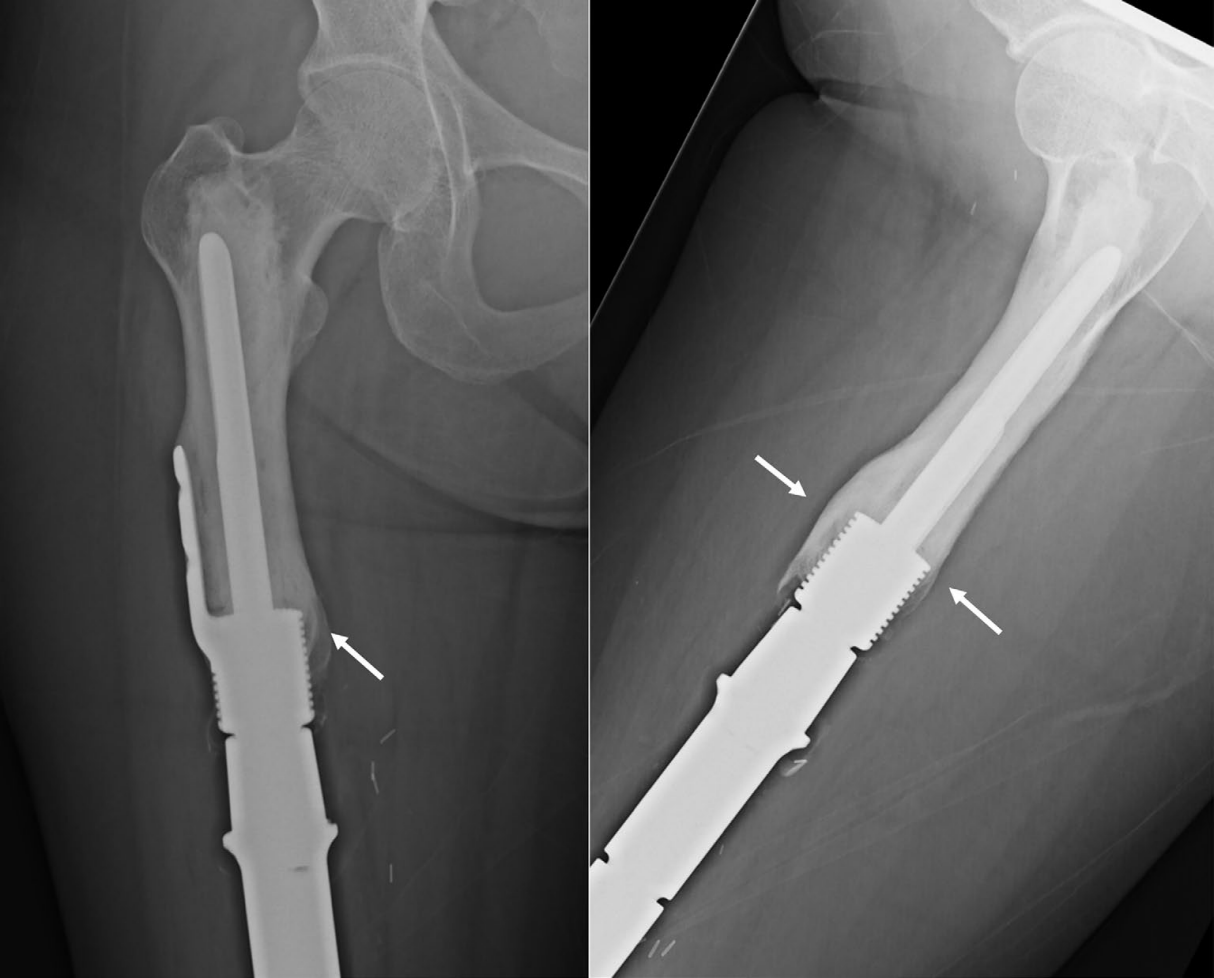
Most recent plain radiographs of patient in Figure 5 showing extracortical bone bridge ingrowth (white arrows).
